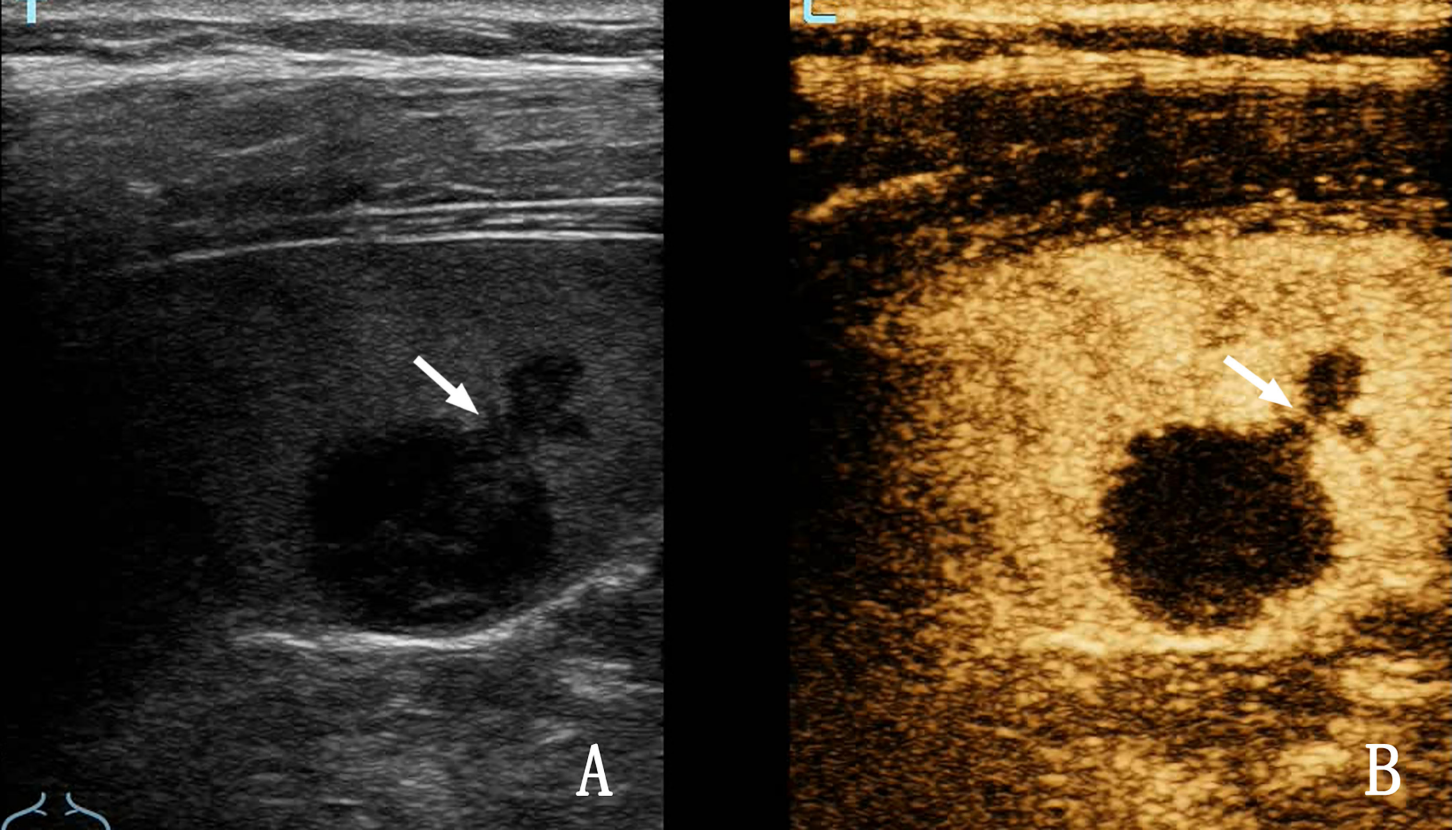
A 32-year-old woman with splenic tuberculosis. (A) Two-dimensional ultrasound (2D US) shows multiple nodules in the splenic region. (B) Contrast-enhanced ultrasound (CEUS) shows that two nodules are conglomerated (arrows), both of which are non-enhanced.
